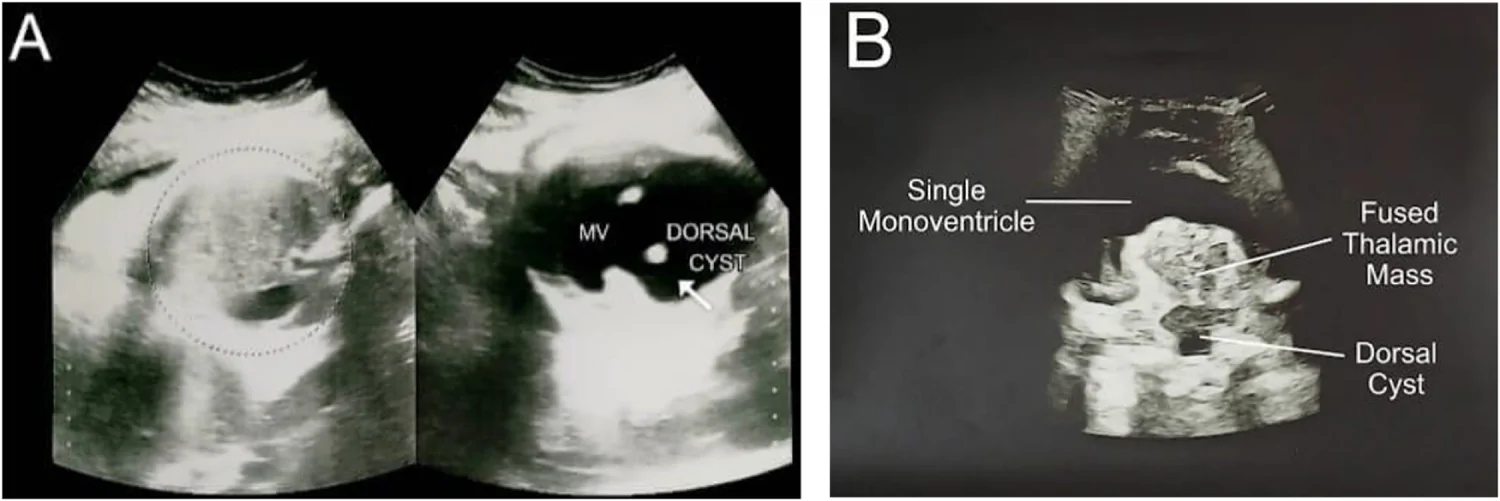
Antenatal and postnatal ultrasonography. (A) Antenatal anomaly scan at 28 weeks’ gestation demonstrating abnormal cranial architecture suggestive of semilobar holoprosencephaly. (B) Postnatal cranial ultrasound confirming a large single midline monoventricle, fused thalami, and a partially formed interhemispheric fissure.
